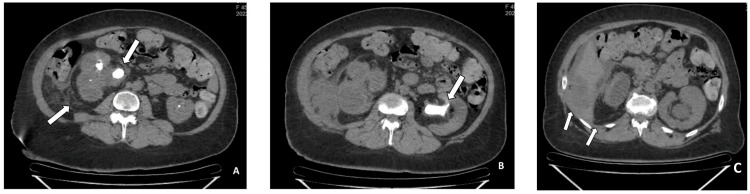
(CT scan demonstrating A) right hydronephrosis due to an obstructive stone with perirenal fat infiltration, (B) left hydronephrosis due to obstructive pyelo-ureteral junction (PUJ) stone, (C) hepatic abscess of segment IV extending into the perihepatic region and the adjacent retroperitoneal space
